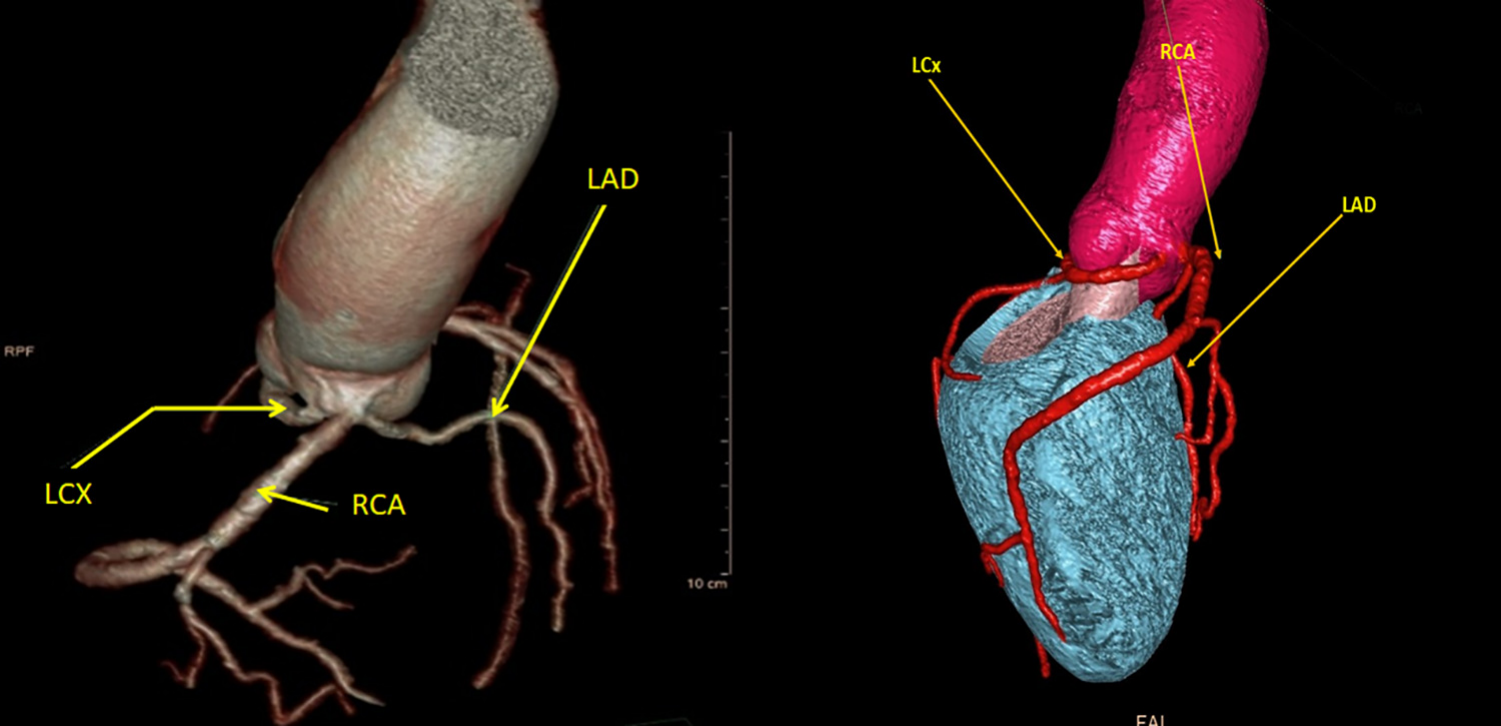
Multi-slice computed tomography (MSCT) of the coronary arteries. All three coronary arteries emerge from right sinus of Valsalva. RCA and LAD emerge from same ostium and lay in close proximity. LCX is positioned laterally and emerges from isolated ostia.
